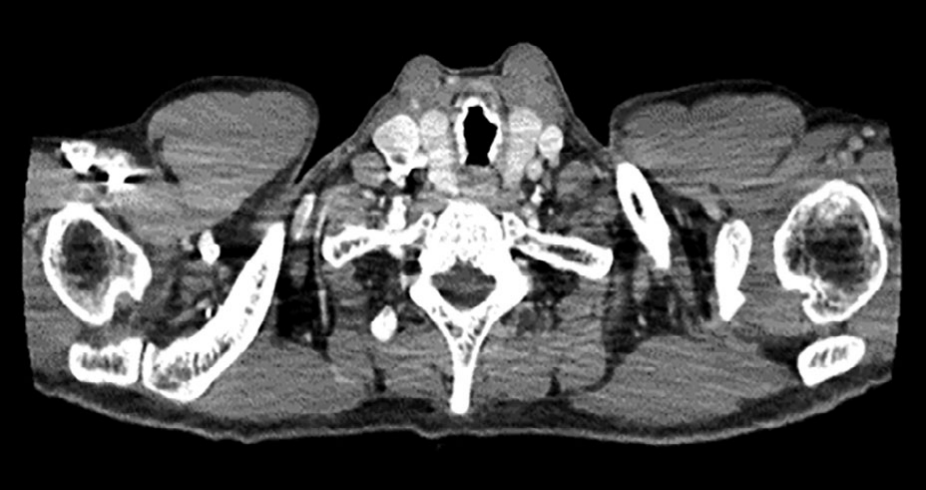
Computed tomography (CT) of the thorax showed variable degrees of stenosis and nodular calcified excrescences were seen protruding into the lumen of trachea.
